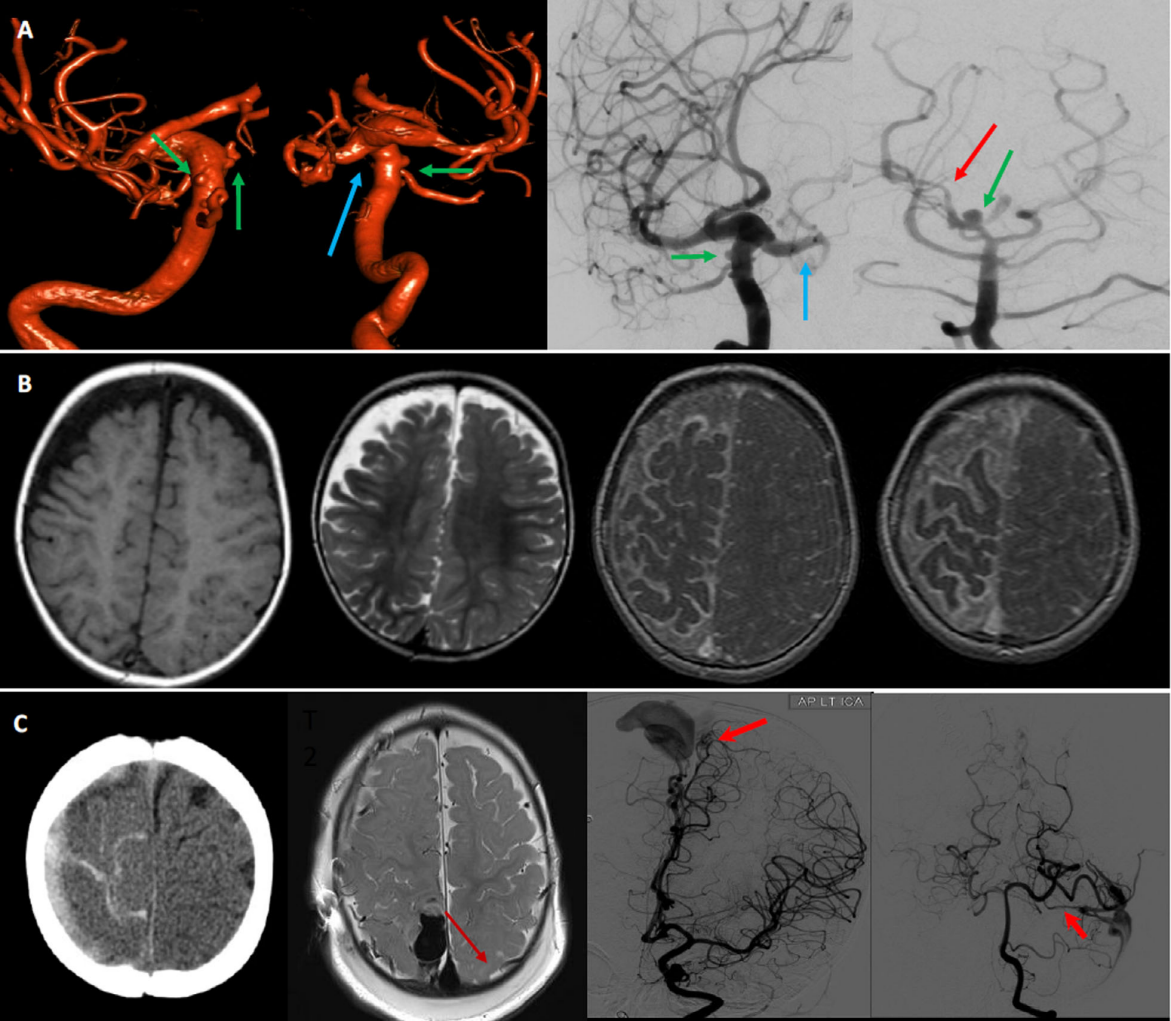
A: 14‐year‐old girl with evidence for arterial abnormalities on standard screening studies. 3D reconstruction of angiography from a right internal carotid artery injection demonstrating multiple small aneurysms (green arrows) arising from a dysplastic appearing supraclinoid region. In addition, focal dysplastic widening of the right posterior communicating artery (blue arrow), small right posterior cerebral artery aneurysm (green arrow), and dysplastic narrowing of the right posterior cerebral artery were observed. B: A 4‐year‐old patient with Sturge‐Weber syndrome. Brain MRI demonstrates asymmetric volume loss (T1), calcification (T2 signal), and abnormal enhancement (pial angiomatosis) in the sulci and surface of the right cerebral hemisphere (T1 postcontrast). C: A previously healthy toddler with acute onset apnea and choking. Noncontrast CT scan demonstrating subarachnoid hemorrhage and subdural hematoma. Axial T2W MRI brain, with abnormal vascular structure (recipient venous pouch) along medial margin of right parietal lobe (arrow). Aangiography demonstrating the enlarged right anterior cerebral artery supplying the right parietal arteriovenous shunt with dilated recipient venous pouch (arrows). AP left vertebral angiogram demonstrating a separate posterior fossa arteriovenous shunt (arrow).
